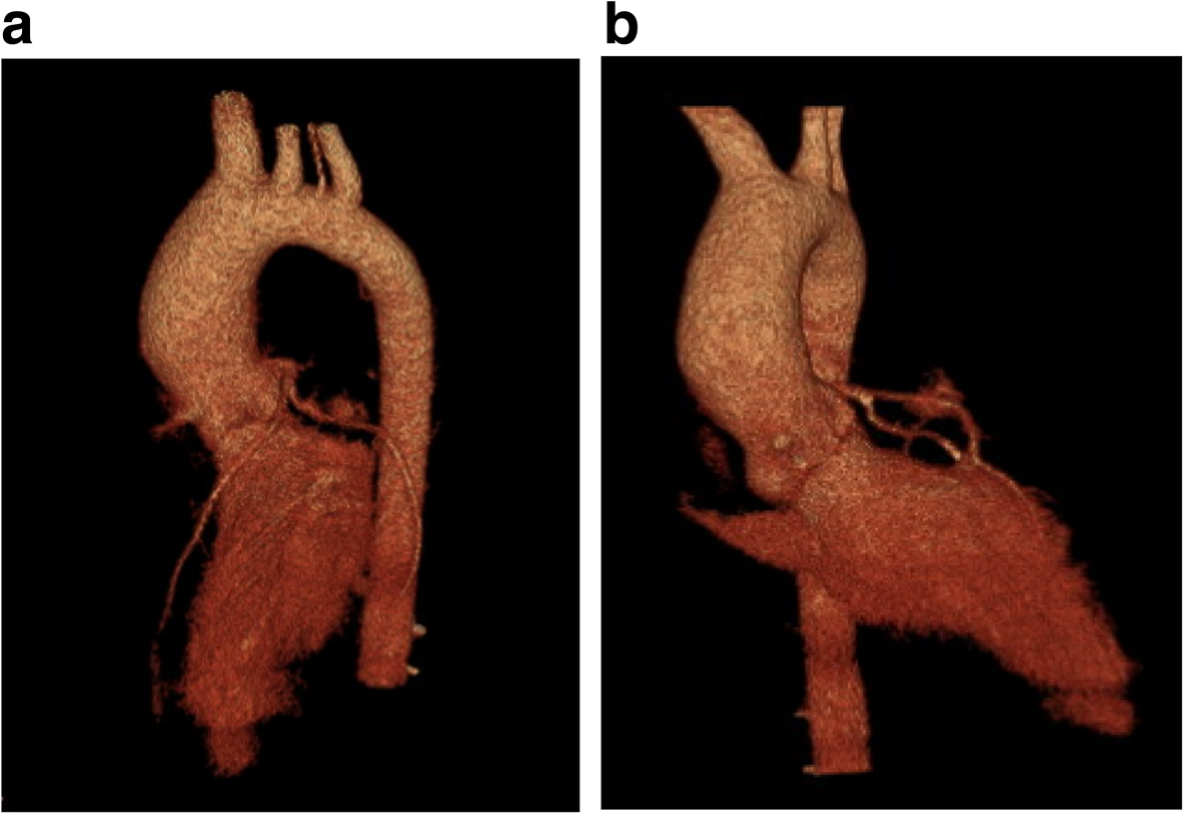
a Lateral and b anterior projection of a three-dimensional reconstructed CT angiography demonstrating a slightly dilated ascending aorta and normal sized aortic annulus
